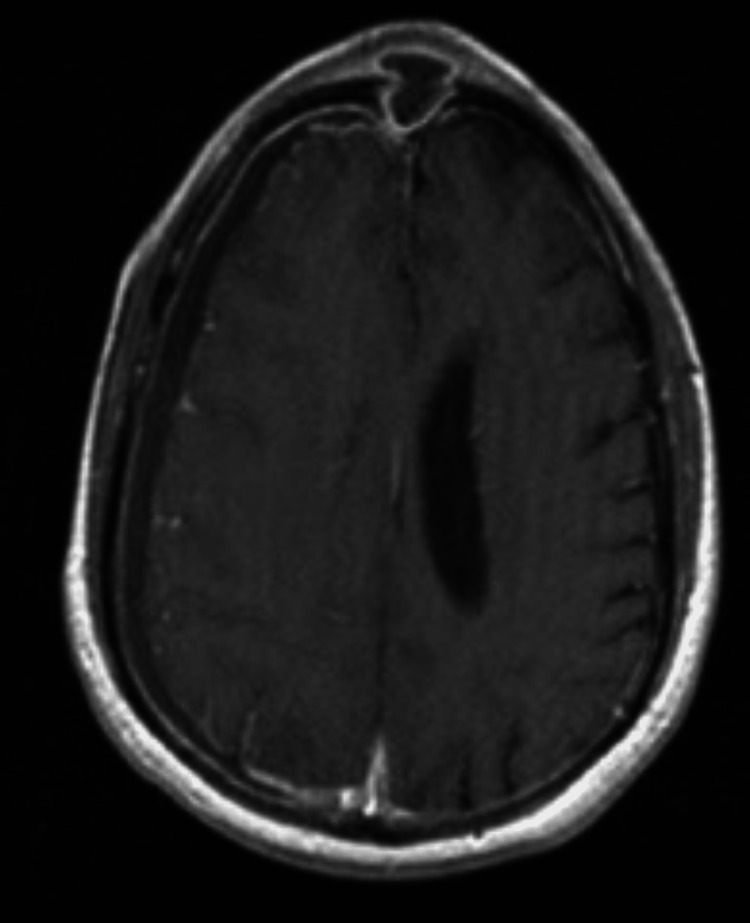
Axial T1-weighted post-gadolinium demonstrating hypointense fluid collection with an enhancement of walls centered at the frontal sinus and extending subcutaneously.
